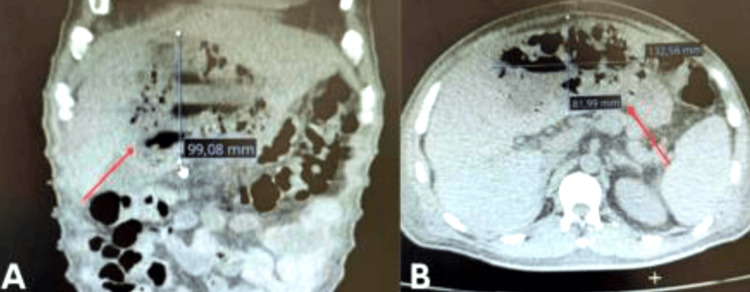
Contrast-enhanced abdominal computed tomography in coronal (A) and axial (B) views showing a multiloculated, gas-forming liver abscess, with a maximum diameter of 13.2 × 9.9 × 8.1 cm. Red arrows indicate the main cavity of the abscess. This finding is consistent with complicated abscesses that require surgical intervention, as in the reported case.
